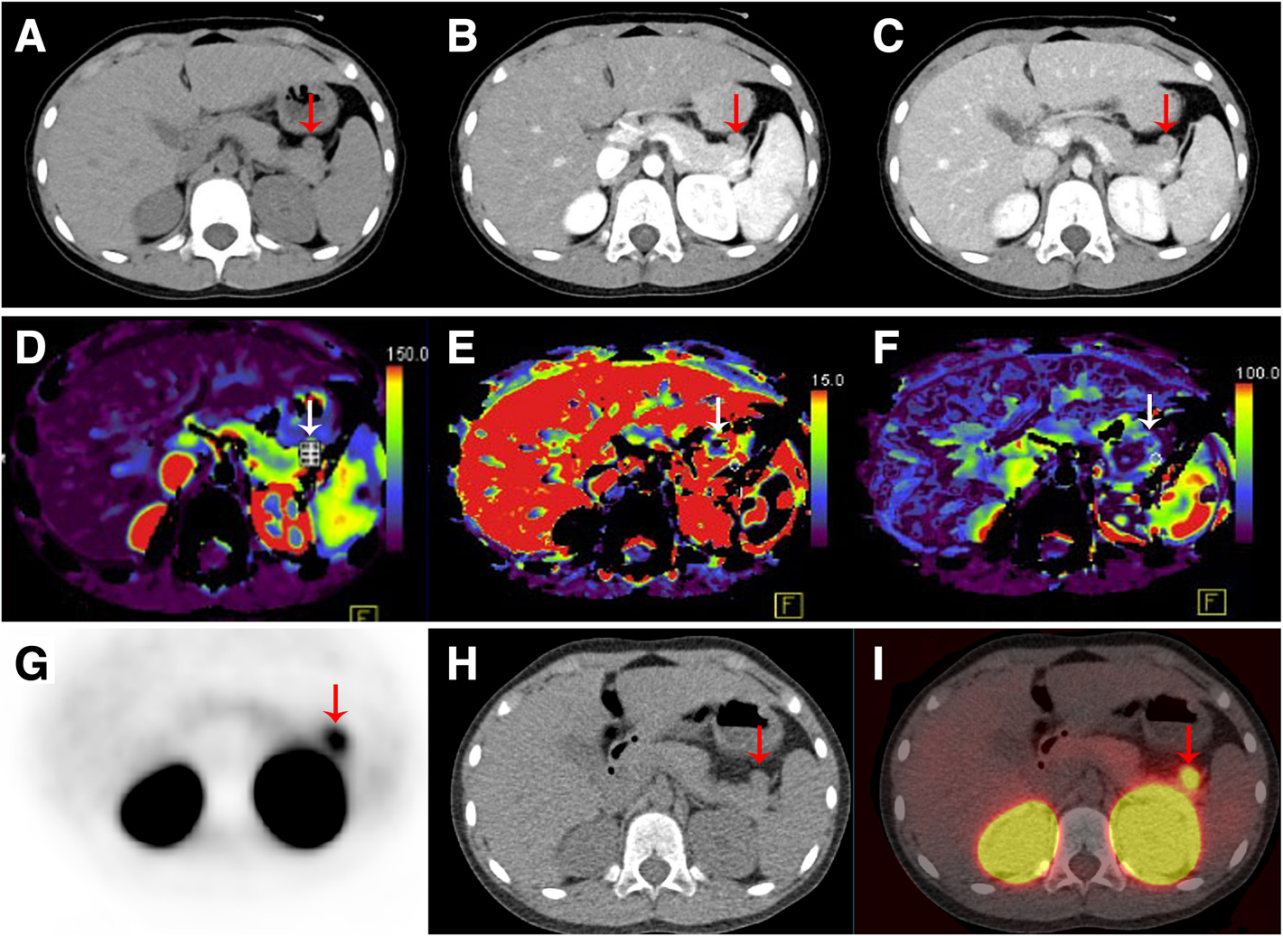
CT and ^68^Ga-exendin-4 PET-CT scan of the pancreas. The tumor is marked with asterisks. a Non-enhanced phase scan. b Arterial phase. c Portal phase. d-f Pancreatic perfusion imaging. g-i
